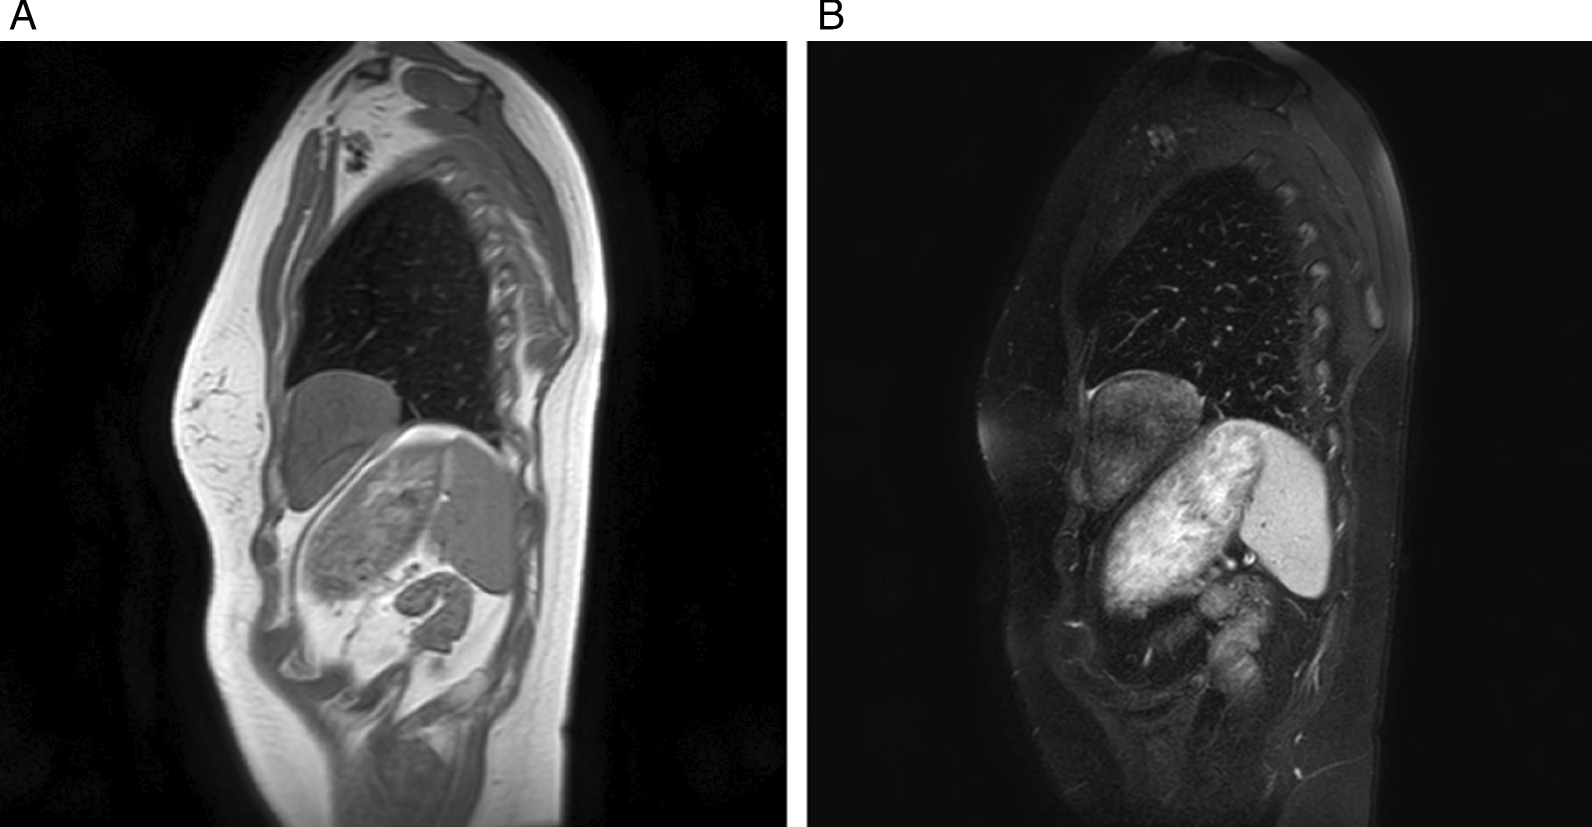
Magnetic resonance images of the tumor. A T1-weighted image. B T2-weighted fat-saturation image. There was no fat intensity detected inside the tumor
